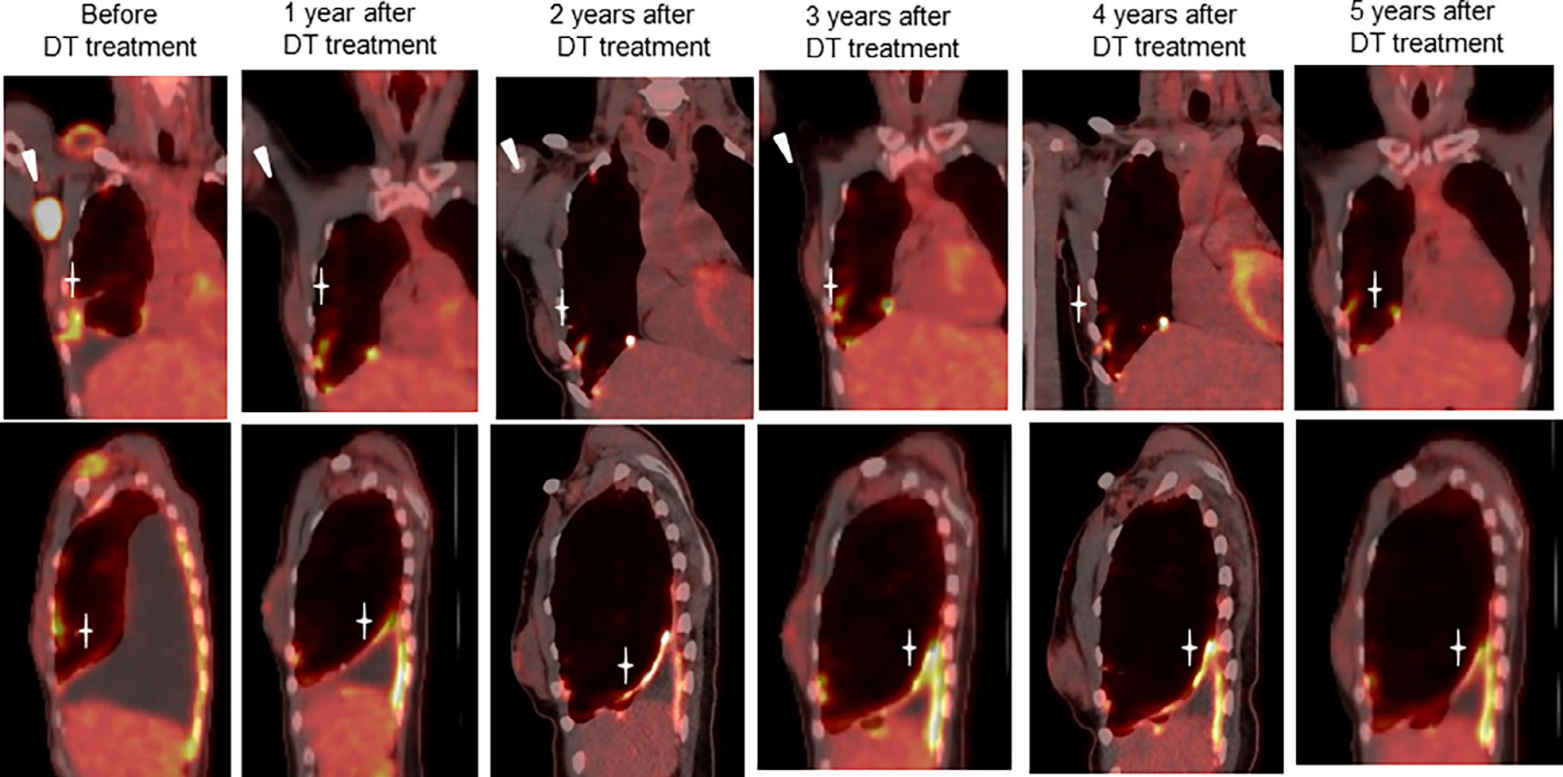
18F‐fluorodeoxyglucose positron emission tomography (18F‐FDG‐PET) images before the start of dabrafenib‐trametinib (DT) treatment showed strong accumulation of FDG in the axillary lymph nodes and diffuse accumulation of FDG on the pleura. After 1 year of treatment, the abnormal accumulation in the axillary lymph nodes disappeared, but the diffuse pleural accumulation repeatedly worsened and decreased.
